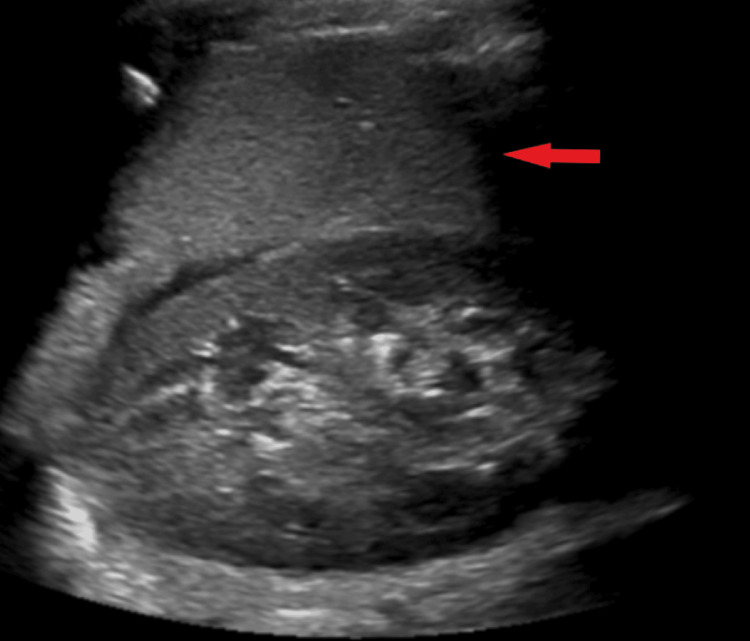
The spleen was measured at 14.2 cm in craniocaudal dimension on abdominal ultrasound corresponding with mild splenomegaly (red arrow).
